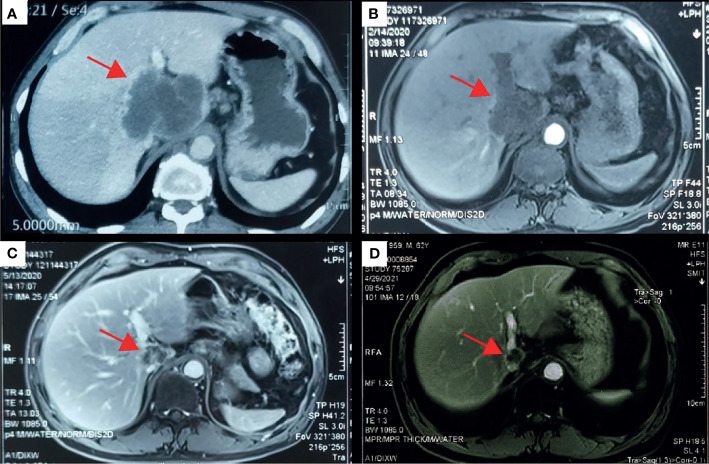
Computed tomography (CT) scans revealed the changes of the primary lesion over time. (A) CT results (December 5, 2019) before first-line therapy (sintilimab combined with gemcitabine plus cisplatin). (B) CT results (February 14, 2020) at the first imaging evaluation. (C) CT results (May 13, 2020) at the end of the first-line systemic therapy. (D) CT results (April 19, 2021) at the latest imaging evaluation of the maintenance therapy.
